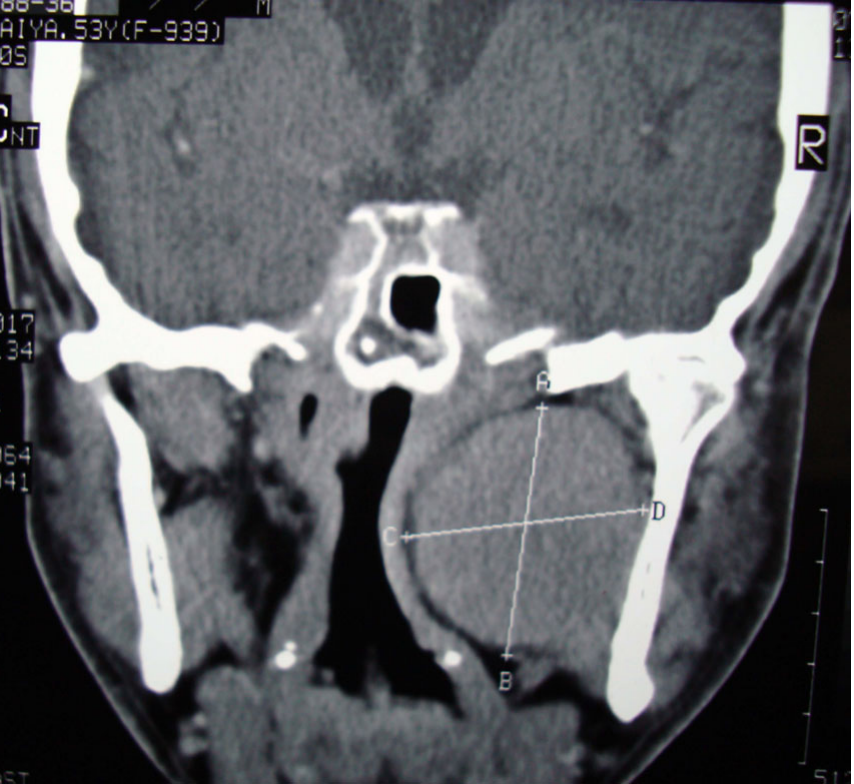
Post contrast coronal CT scan showing parapharngeal lesion.
